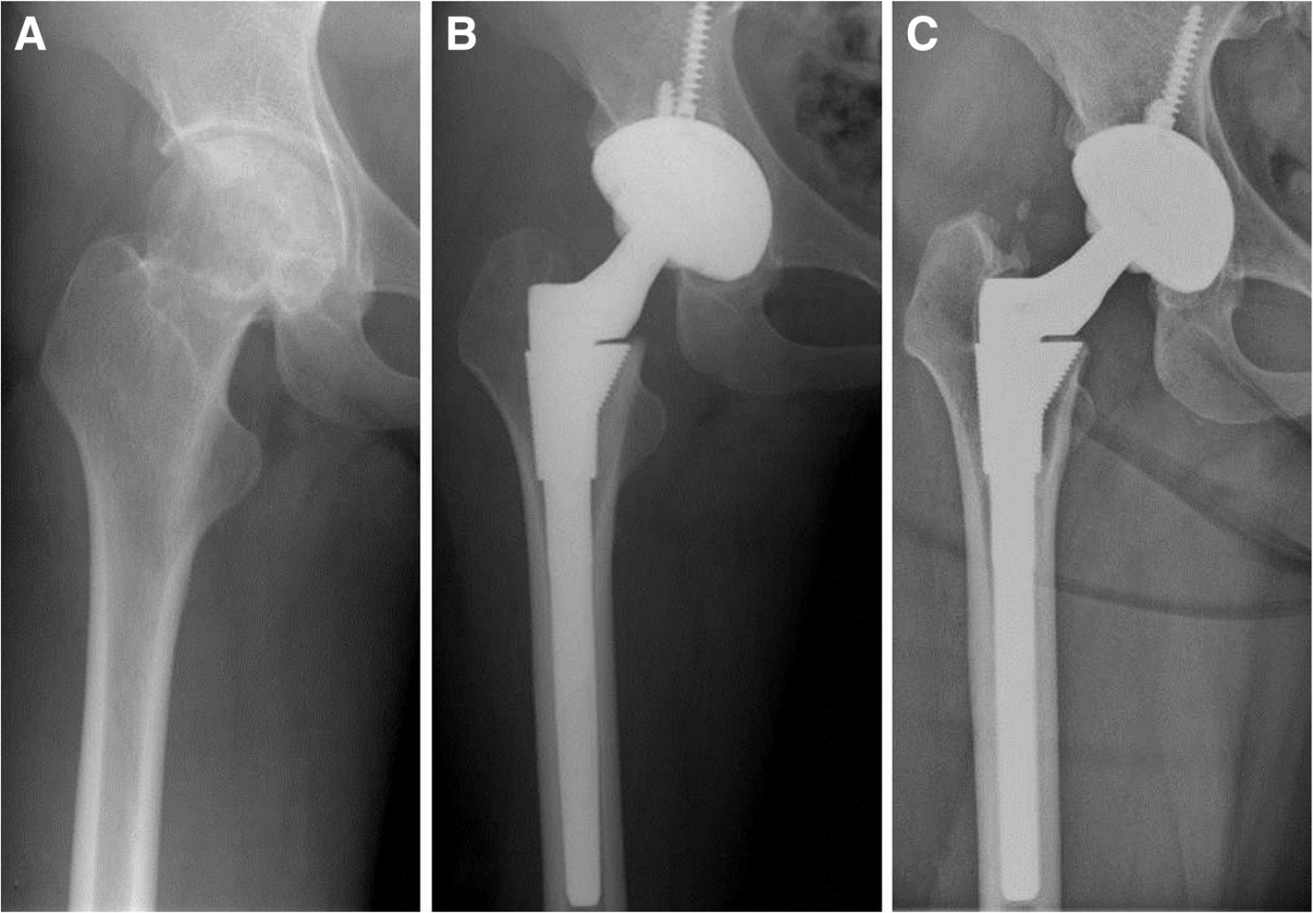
a Pre-operative radiograph showed osteonecrosis of the femoral head in patient with primary immune thrombocytopenia. b Preoperative transfusions of six units of platelet concentrate and two units of packed red blood cells were performed. Postoperatively, six units of platelet concentrates and four units of packed red blood cells were transfused. Post-operative radiograph of cementless total hip arthroplasty performed with ceramic-on-ceramic bearing. c Twelve-year follow-up radiograph showed a well-fixed prosthesis with no osteolysis
